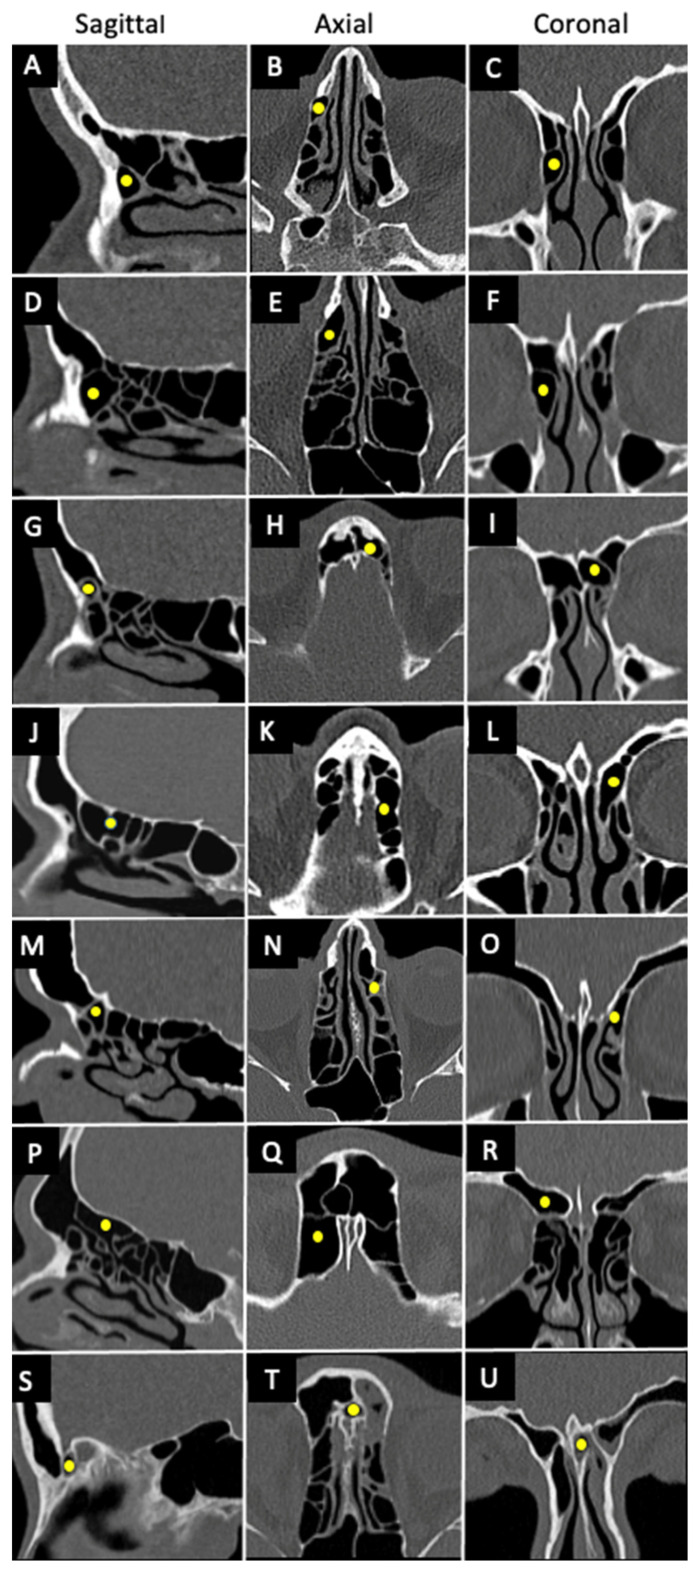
IFAC cell types using computed tomography. Different cell types according to IFAC on CT using sagittal, axial and coronal planes for each cell type: (A–C) aggar nasi cell, (D–F) supra aggar cell, (G–I) supra aggar frontal cell, (J–L) supra bulla cell, (M–O) supra bulla frontal cell, (P–R) supraorbital ethmoid cell, and (S–U) frontal septal cell.
